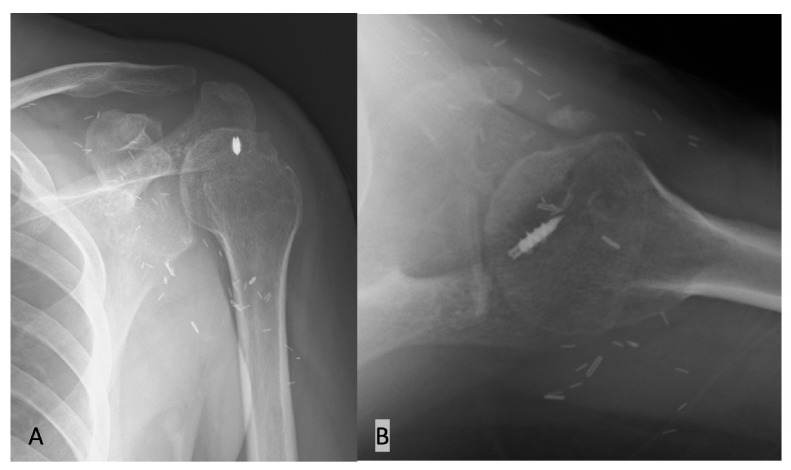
X-ray follow ups at 3 months after surgery. (A) Anteroposterior view. (B) Axillary view.
